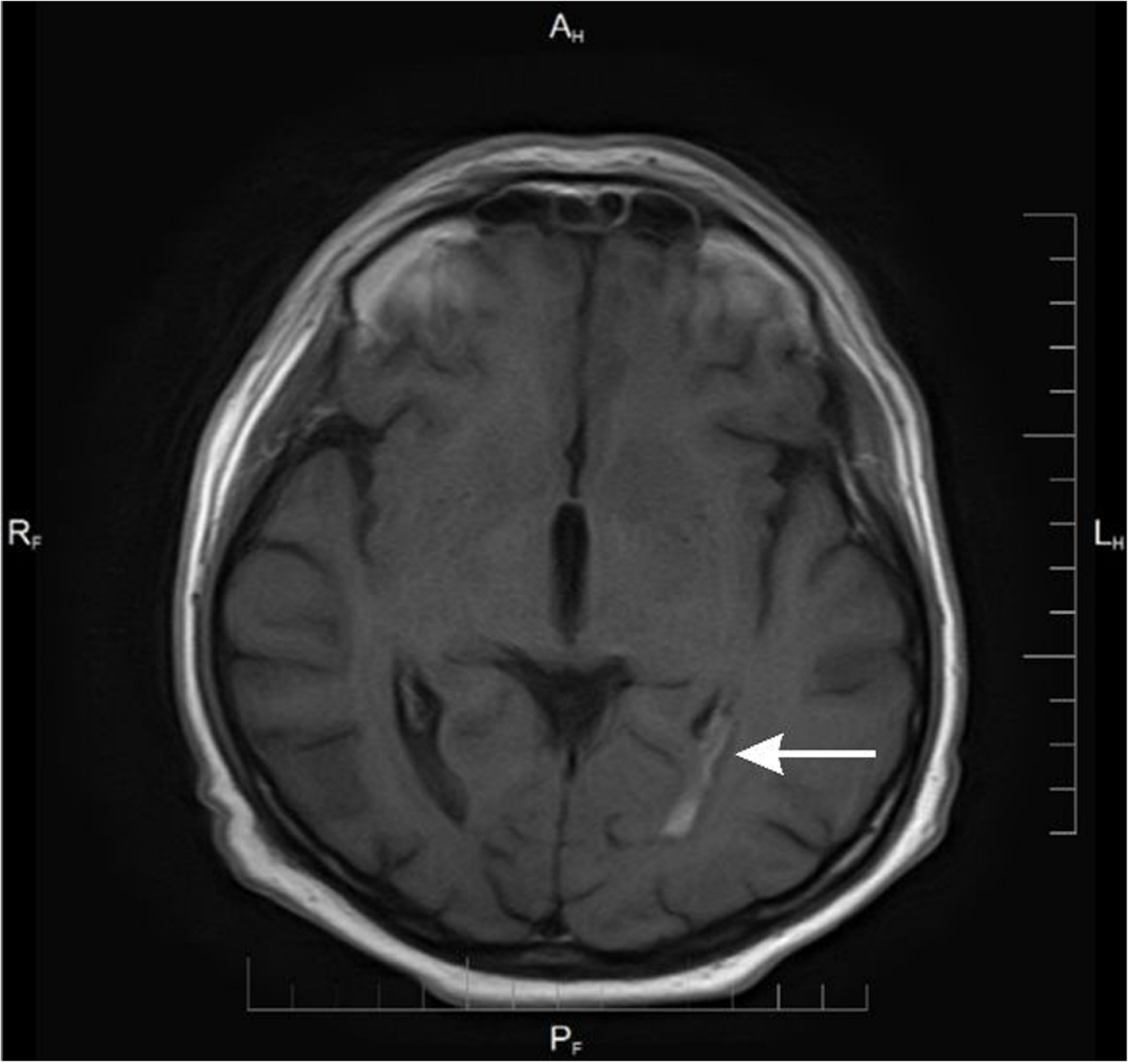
Axial T1-weighted scan after contrast enhancement - Enhancing lesion in choroid plexus of the left occipital temporal horn of the lateral ventricle with surrounding edema visible in FLAIR scan
